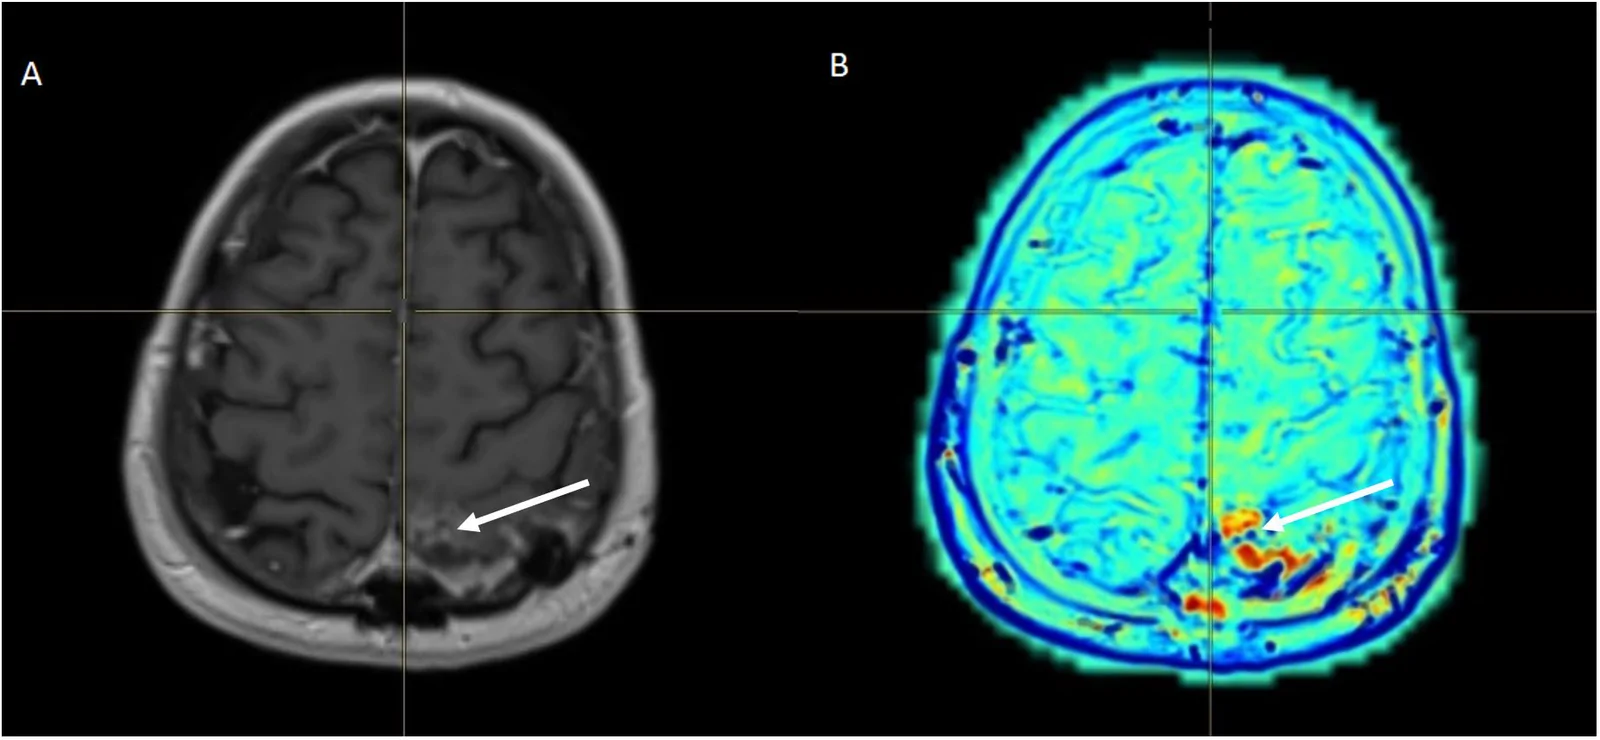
Post-operative TRAMs/CCA findings demonstrating focal contrast clearance adjacent to areas of delay contrast retention with the contrast-enhancing tissue surrounding the resection cavity. (A) Contrast-enhanced T1-weighted MRI performed 25 days after resection for SRS planning demonstrating equivocal post-operative contrast enhancement. (B) TRAMs/CCA demonstrating focal contrast clearance adjacent to areas of delayed contrast retention.
